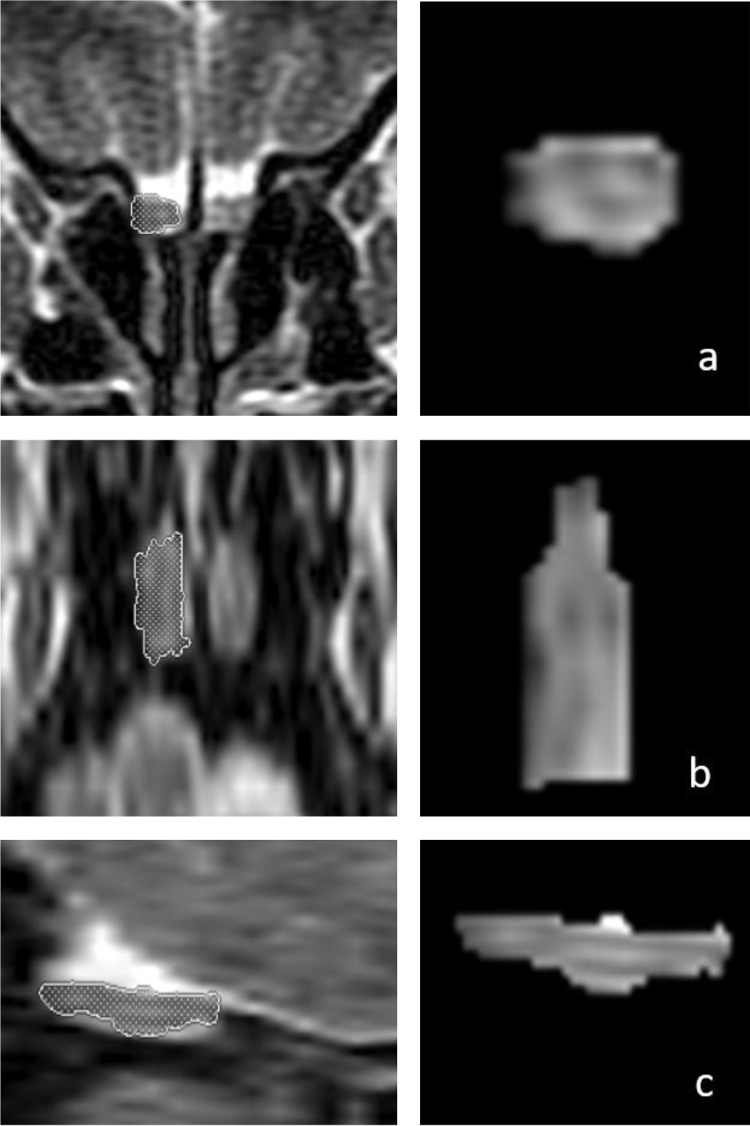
Three-dimensional measurements of the right olfactory bulb (OB) volume in a 66-year-old male patient on T2-weighted coronal (a, first row), axial (b, middle row), and sagittal (c, bottom row) magnetic resonance images. The patient’s right OB volume was 76 mm^3^ after olfactory rehabilitation.
